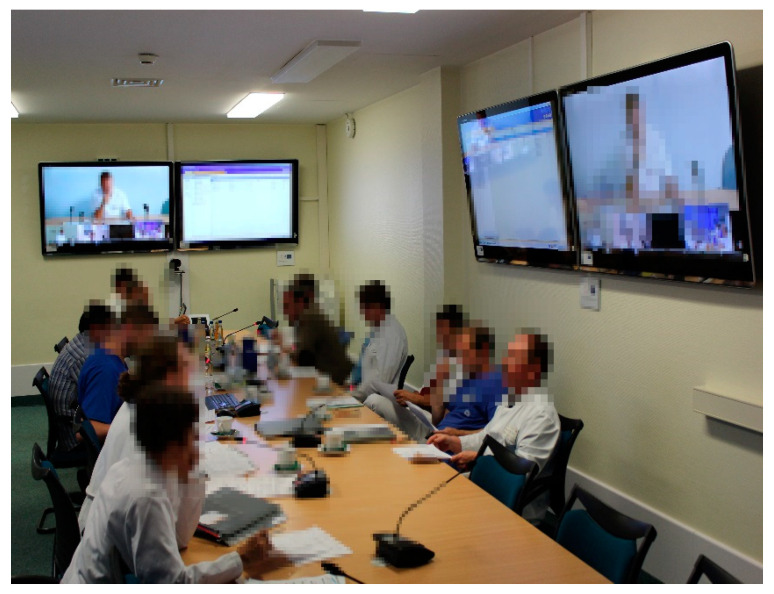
Tele-Tumour Conferencing. A sophisticated subproject included the tumour board of Eberswalde hospital, where smaller hospitals presented their cancer patients to specialists. Seated at the conference table are oncologists, a radiation oncologist and a radiologist. A pathologist in Pasewalk is discussing cases with a referring physician from the Templin hospital, approximately 65 km away. Documents, X-rays and pathology slides can be viewed by all participants simultaneously.
